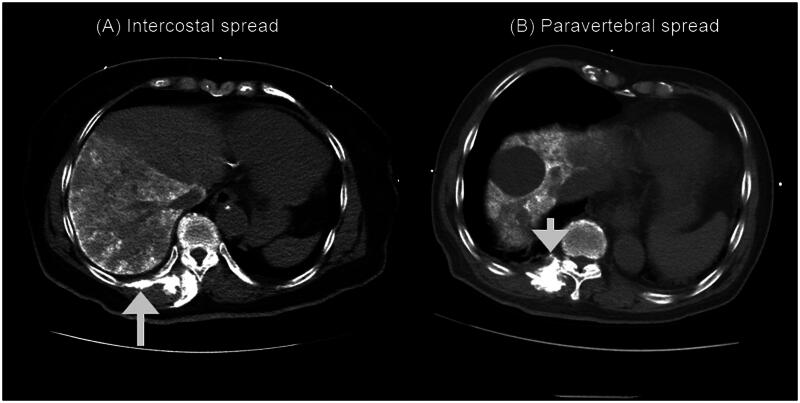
Computed tomography scan of the spine showing injectate spread. (A). Intercostal spread (upward arrow). (B). Paravertebral spread (downward arrow).
